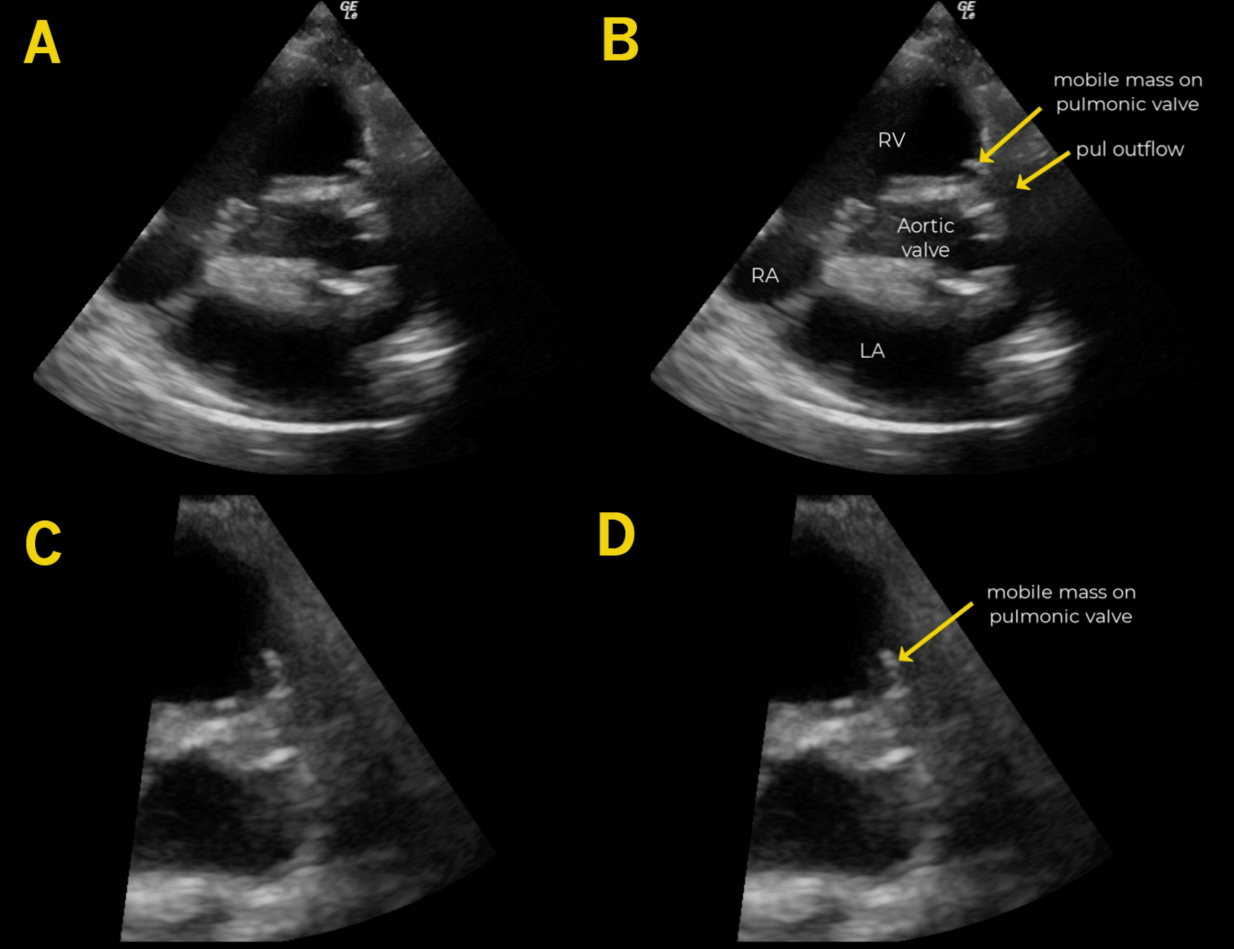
Pulmonic Valve Vegetation In these parasternal short-axis views taken at approximately the aortic valve level, an echogenic mobile mass (arrow) is seen attached to the pulmonic valve. It is even more prominent in the zoomed-in images (C-D). The right atrium (RA), right ventricle (RV), left atrium (LA), aortic valve, and pulmonic outflow are noted (B).
